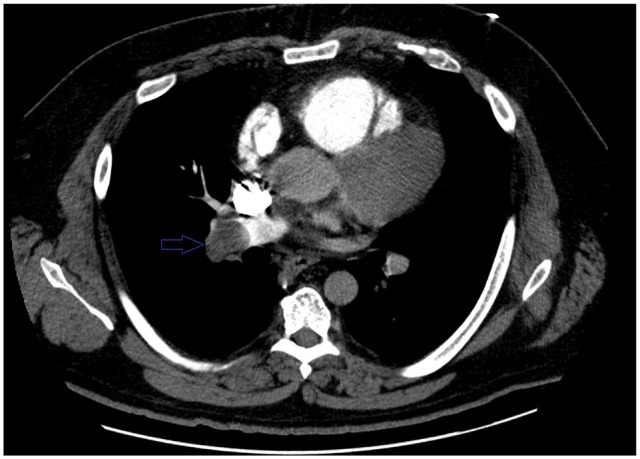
Computed tomography pulmonary embolism (CTPE) showing right pulmonary artery PE.
